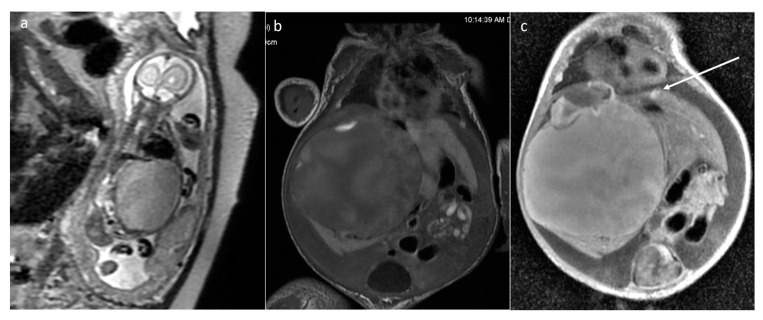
Another case of BRBNS in a fetus. (a) Coronal SSFSE/T2WI. Postnatal MRI of the intra-abdominal mass with peripheral contrast enhancement and hemorrhagic foci (bright spots on T1-weighted images); (b) coronal T1WI; (c) coronal CE-T1WI+fatsat; (arrow points at another small lesion in the left liver lobe).
